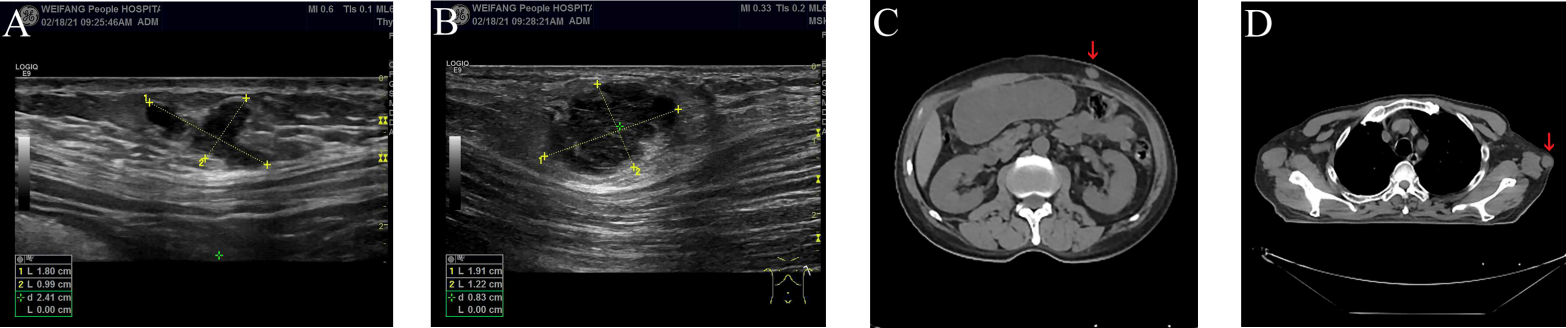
Ultrasound and CT scan of subcutaneous soft tissue metastases (A–D). (A) Ultrasound scan of subcutaneous soft tissue metastasis in the left upper quadrant of the abdomen. (B) Ultrasound scan of subcutaneous soft tissue metastasis in the left posterior thorax. (C) CT scan of subcutaneous soft tissue metastasis in the left upper quadrant of the abdomen (red arrow). (D) CT scan of subcutaneous soft tissue metastasis in the left posterior thorax (red arrow).
